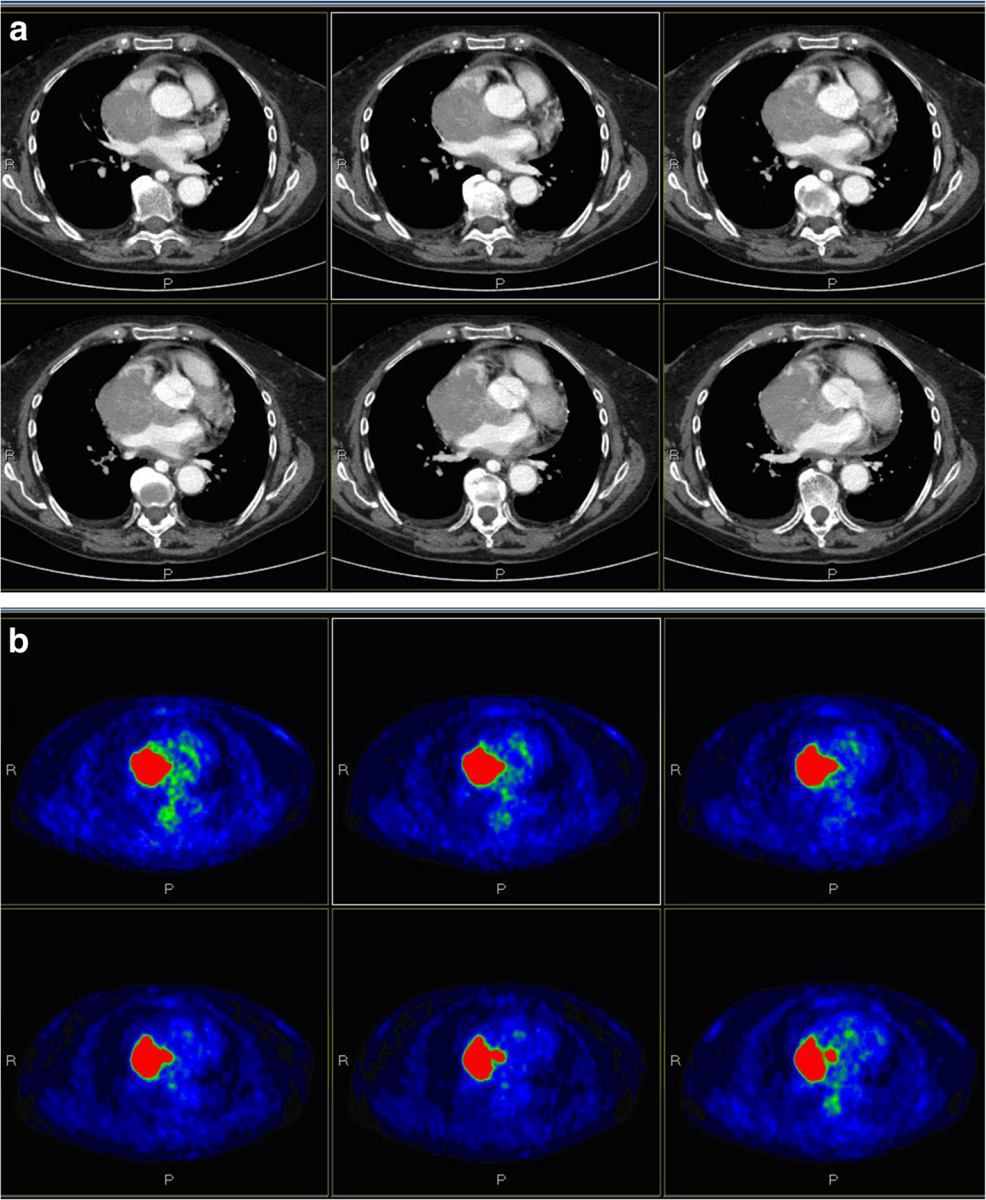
Radiographic images of the mass. ( A) Computed tomography of the chest documenting the atrial mass; ( B) fluorine-18-fluorodeoxyglucose positron emission tomography confirming this data.
